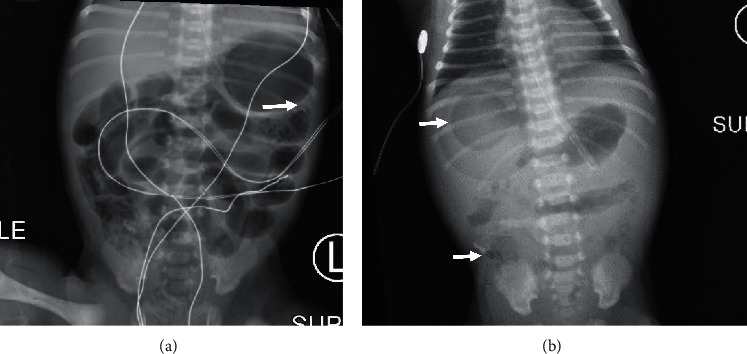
Abdominal x-rays of the premature infant. (a) Image obtained at 30 h of age. Arrow indicates the area of pneumatosis intestinalis. (b) Image obtained at 36 h of age. Upper arrow points to one area of pneumoperitoneum, and the lower arrow points to an area of pneumatosis intestinalis.
